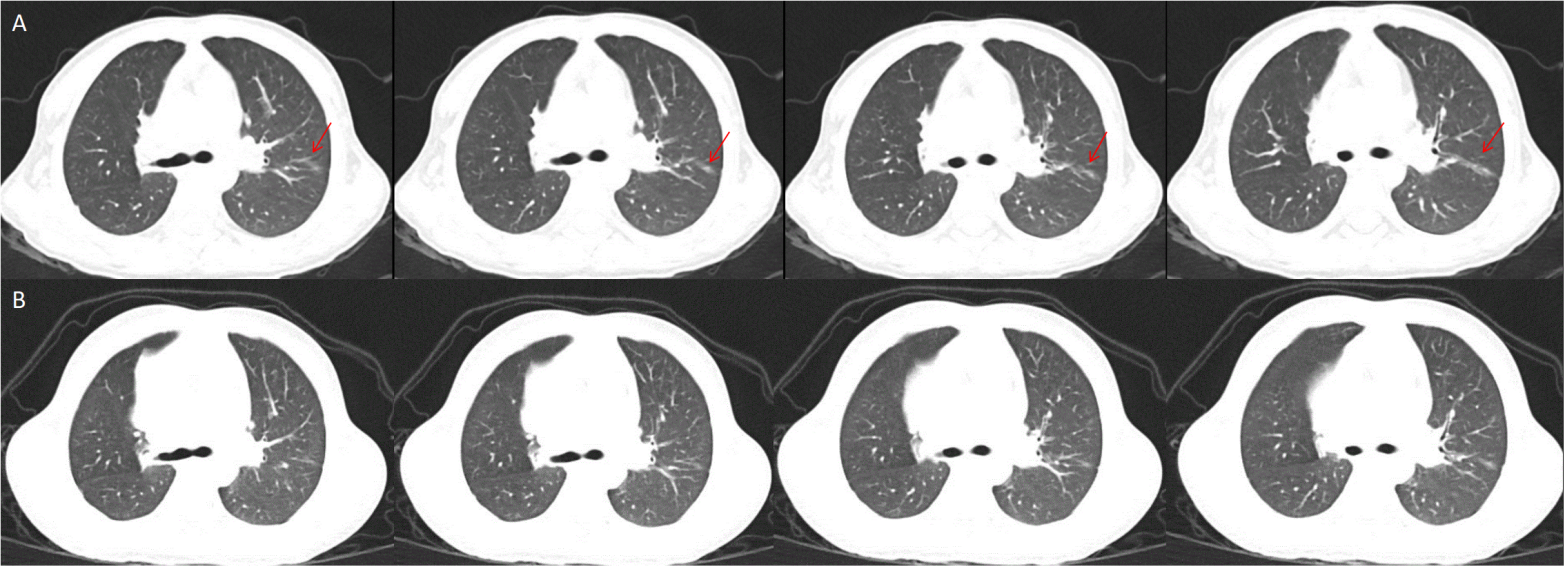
Interstitial lung disease in the left lung, the red snip point (A), Pulmonary CT after eight months of Canakinumab treatment (B).
